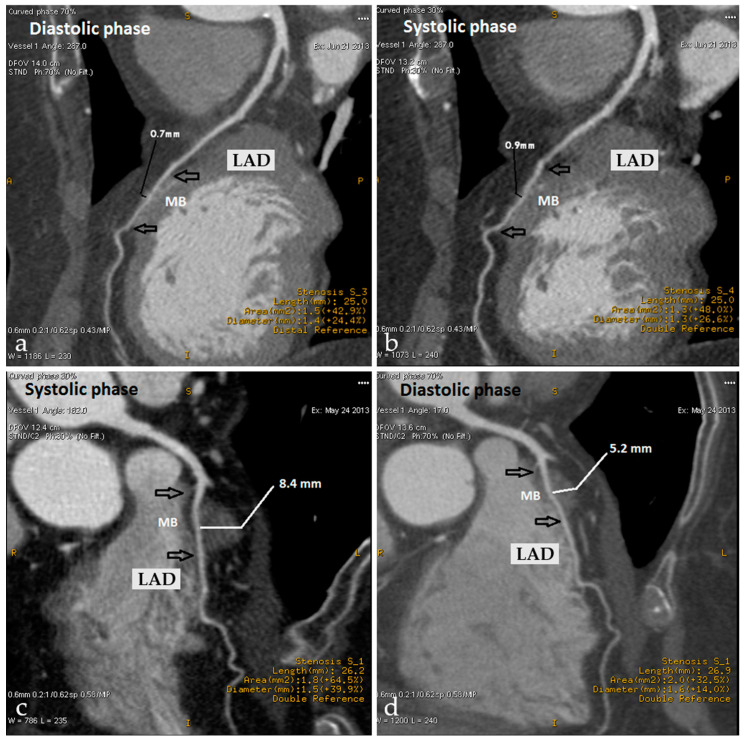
Cardiac computed tomography images of mid-diastolic (a) and end-systolic (b) phases showing very thin MB at middle LAD without significant systolic compression (arrows). Significantly thicker MB at middle LAD (c,d), demonstrating systolic compression of the tunneled artery (arrows).
